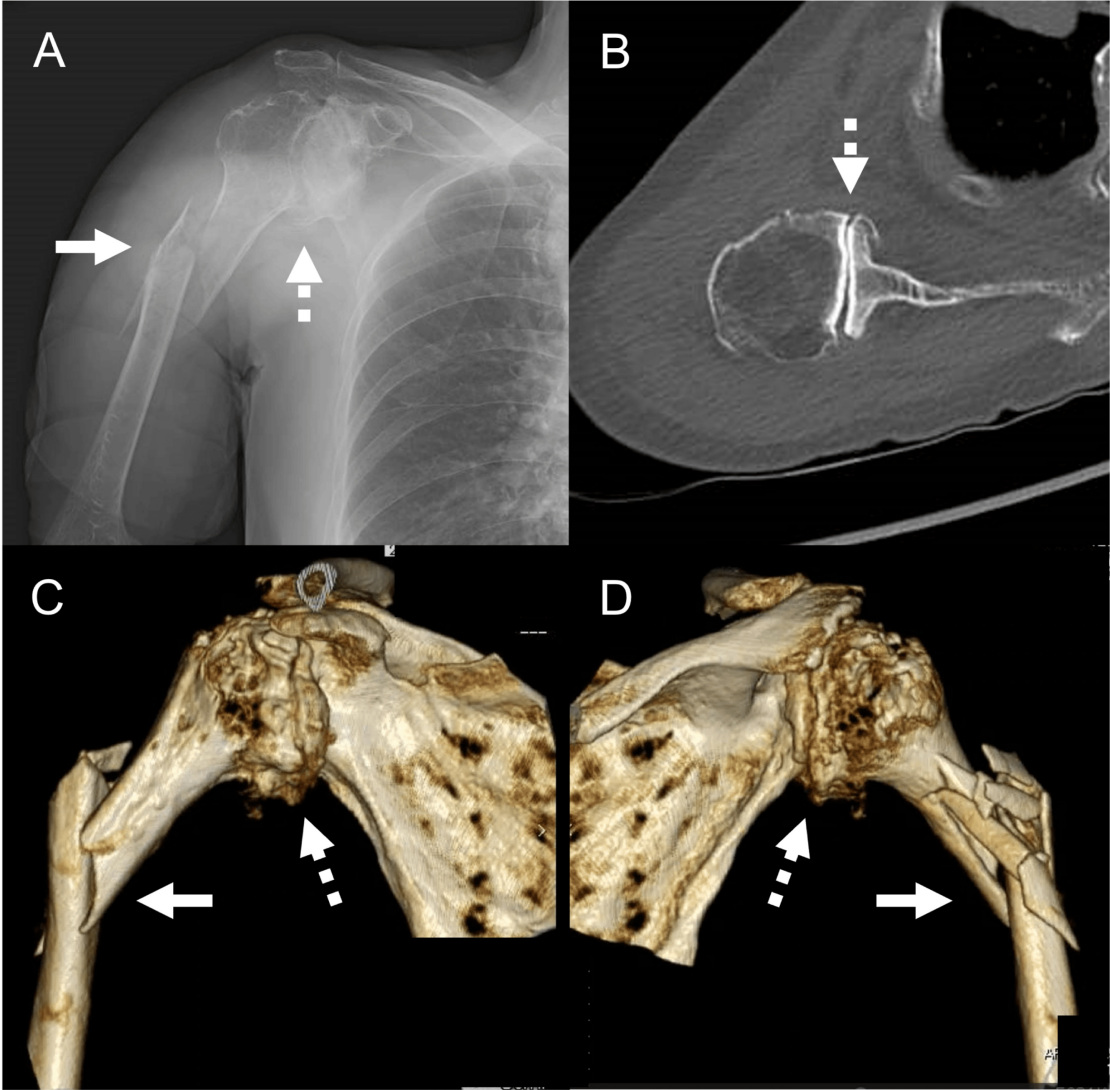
The preoperative images of the right shoulder. The preoperative images of the right shoulder include anteroposterior X-ray (A), axial view of CT (B), anterior view of 3D-CT (C), and posterior view of 3D-CT (D). These images show a metadiaphyseal humeral fracture (indicated by an arrow) with severe osteoarthritis (OA) (indicated by a dotted arrow).
